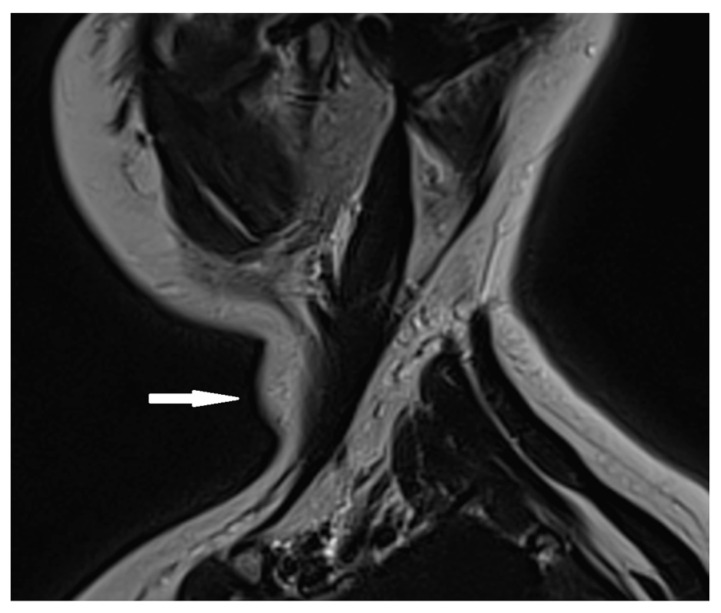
Cervical MRI with gadolinium contrast showing a gadolinium-avid tumor (arrow) located posteriorly to the left thyroid lobe.
